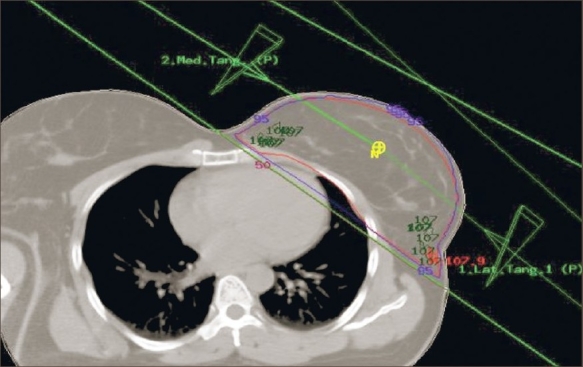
CT-based planning for a target volume of 1,231 cc
